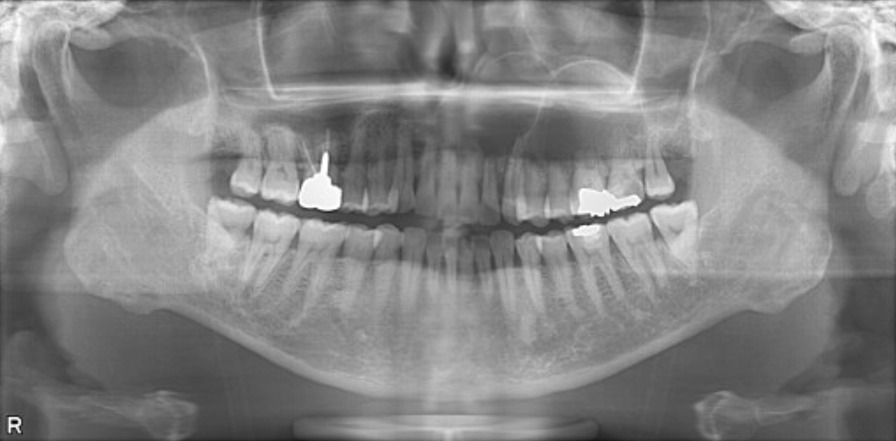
Panoramic X-ray findings at the initial examination. A multilocular cystoid radiolucency was observed around the left maxillary sinus, and knife-cut-like root resorption was noted
